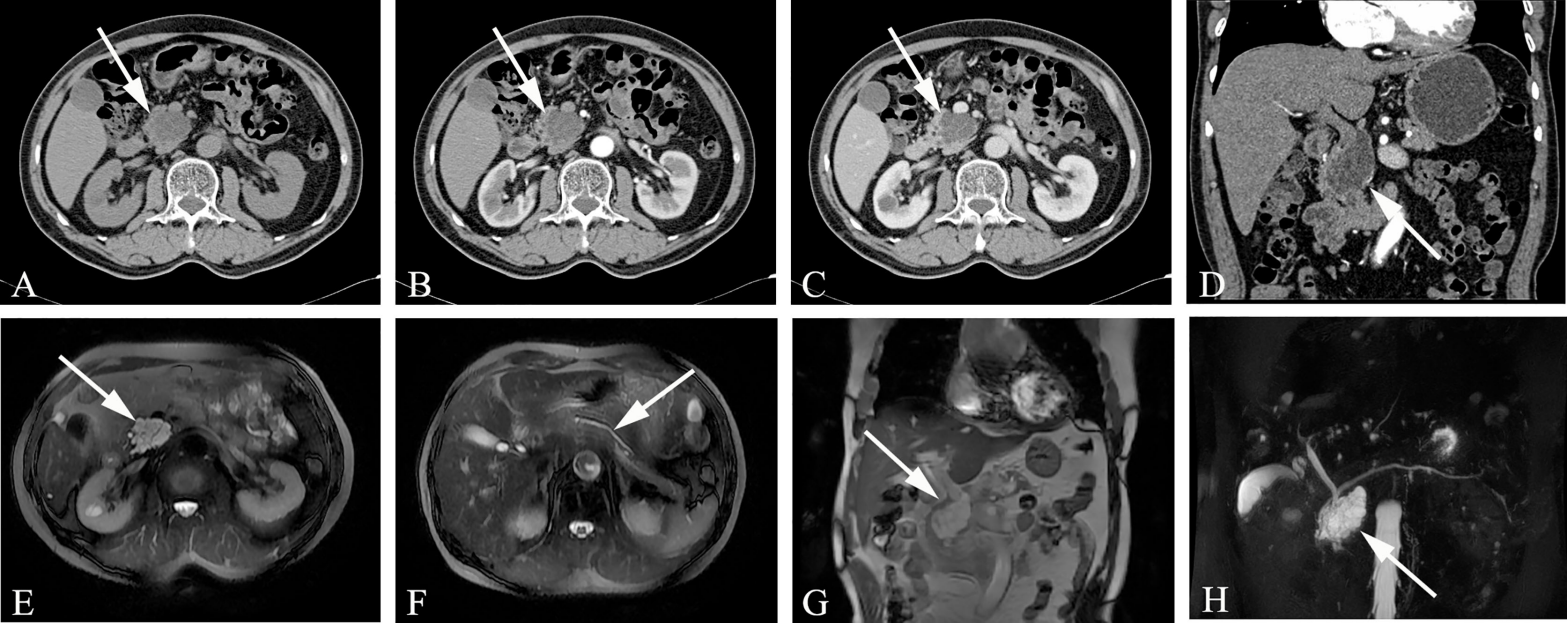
Branch-type IPMN (Male, 66y, physical examination revealed a pancreatic mass for a week). (A–C) The CT plain scan, arterial phase and venous phase at the same level; (D) Coronal image in arterial phase. (E) T_2_-weighted cross-sectional image; (F) T_2_-weighted cross-sectional image at another level of the same patient; (G). T_2_-weighted coronal image; H. MRCP reconstruction map; (A) The CT plain scan showed multilocular cystic mass of pancreatic head with septation and clear boundary. The density is slightly higher than that of water. (B–D) Contrast enhanced scan showed moderate enhancement of mural nodules in the dilated branch pancreatic duct. (E–H) Septa can be seen in the lesion and the MPD was slightly dilated. The white arrows in the Figures only indicate the location of the neoplasm.
